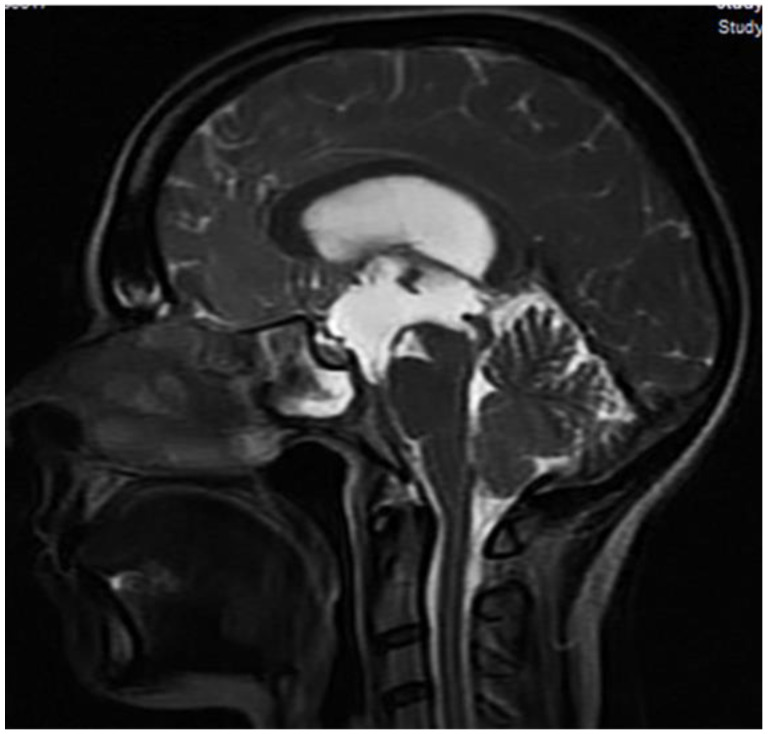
MRI shows Aqueduct stenosis.
